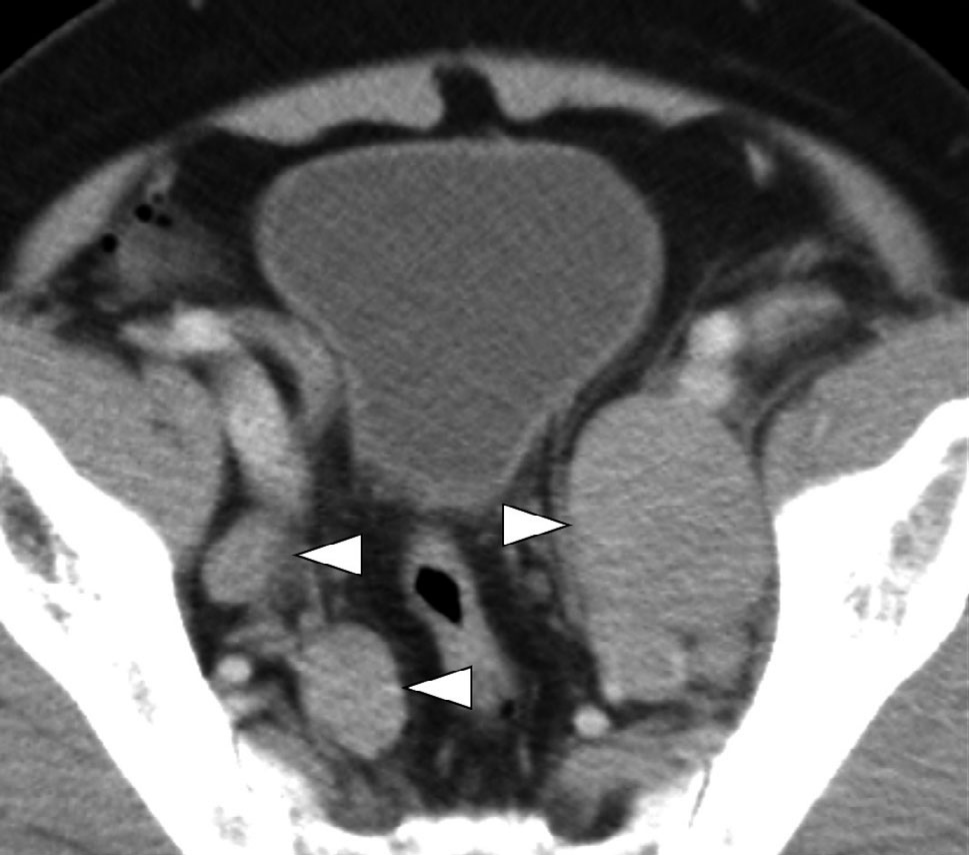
CT image at presentation showing multiple swollen pelvic lymph nodes (arrowheads).
